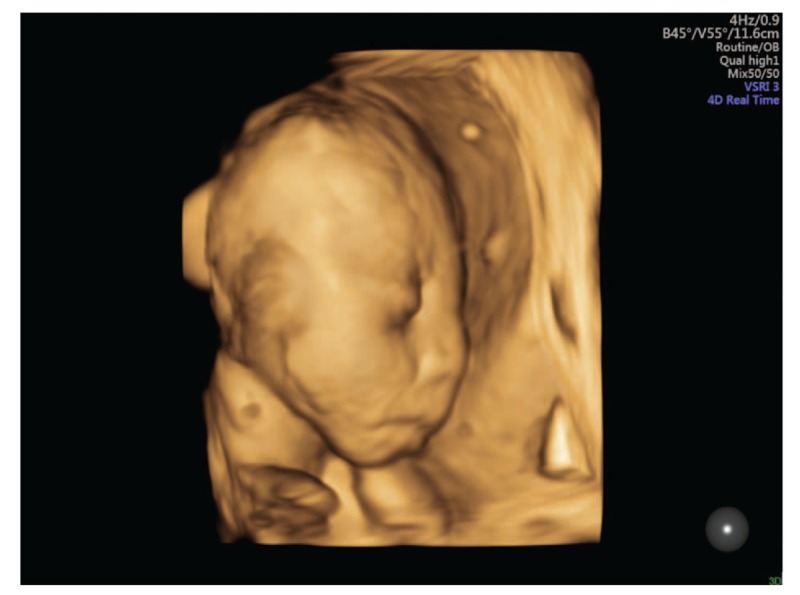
Three-dimensional ultrasound of the fetal facial profile with micrognathia.
